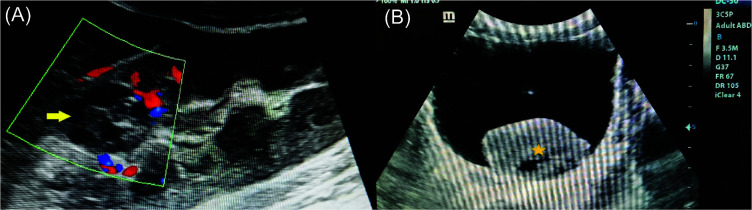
Color Doppler ultrasonography image demonstrating no significant vascularity within the well-circumscribed heteroechoic cortical based lesion (arrow) in the upper pole of right kidney. (A) High-resolution transverse ultrasonography image demonstrating a giant retained clot (star) within the lumen of the urinary bladder secondary to symptomatic hematuria (B).
